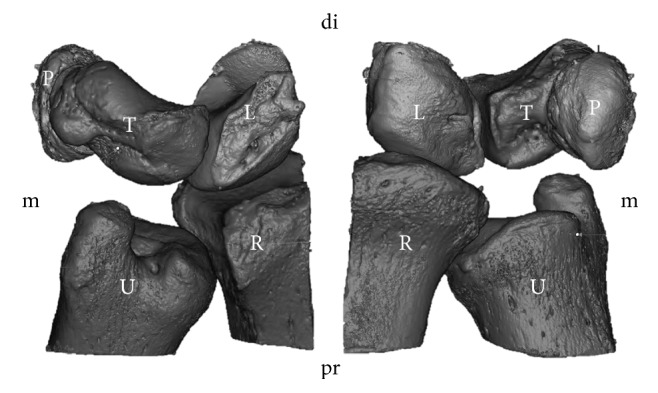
Image-based reconstruction from microcomputed tomography and magnetic resonance imaging. The triangular fibrocartilage complex could not be visualized to sufficient extent using this method.
